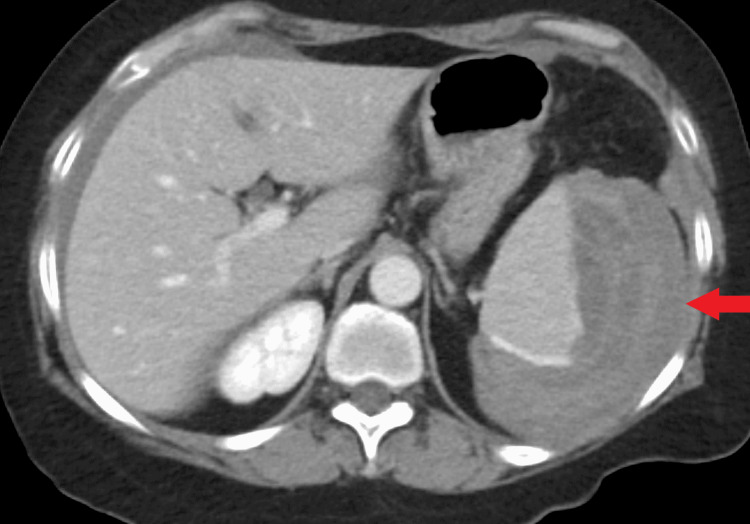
Axial CT abdomen with contrast showing subcapsular perisplenic hematoma (red arrow).
